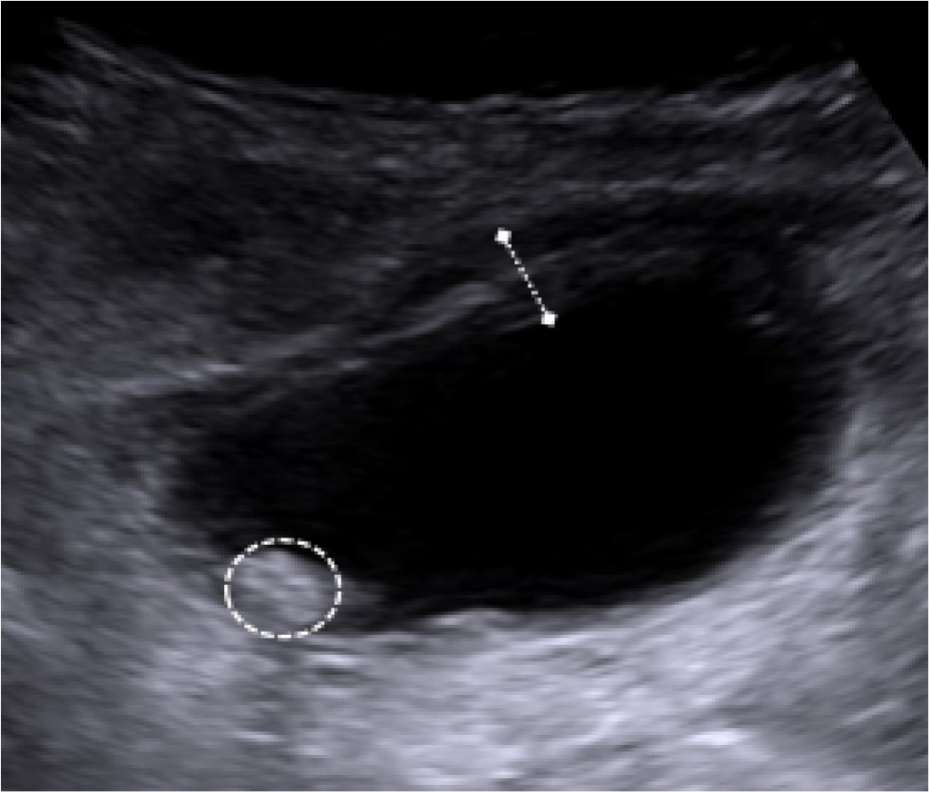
Ultrasound of the gallbladder obtained 1 day post-admission shows a thick-walled gallbladder containing a gallstone (circled).
